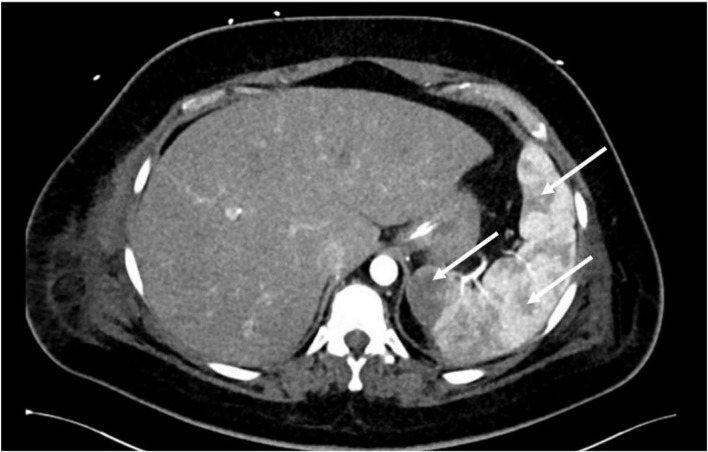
Computed tomography scan revealing splenic infarctions. Computed tomography of the abdomen on post-operative day 17 showed multiple splenic infarctions deemed to be of thromboembolic origin. White arrows indicate exemplary infarctions.
